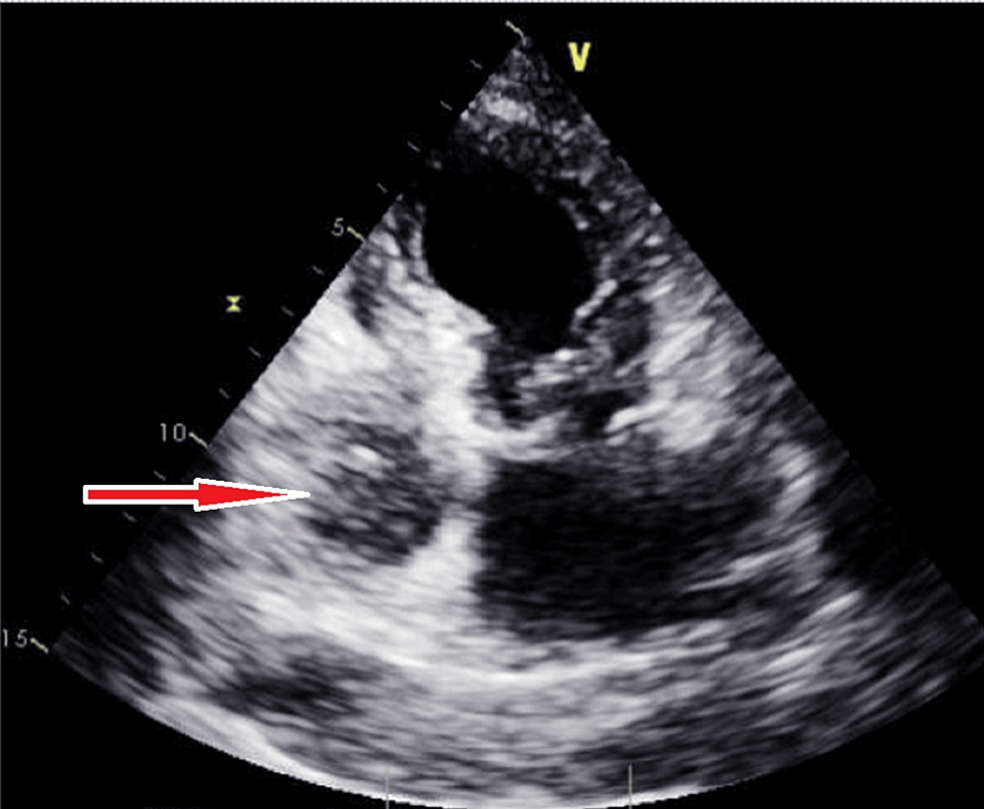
Transthoracic echocardiogram showing the multiloculated hypoechoic mass in the right atrium (arrow).
